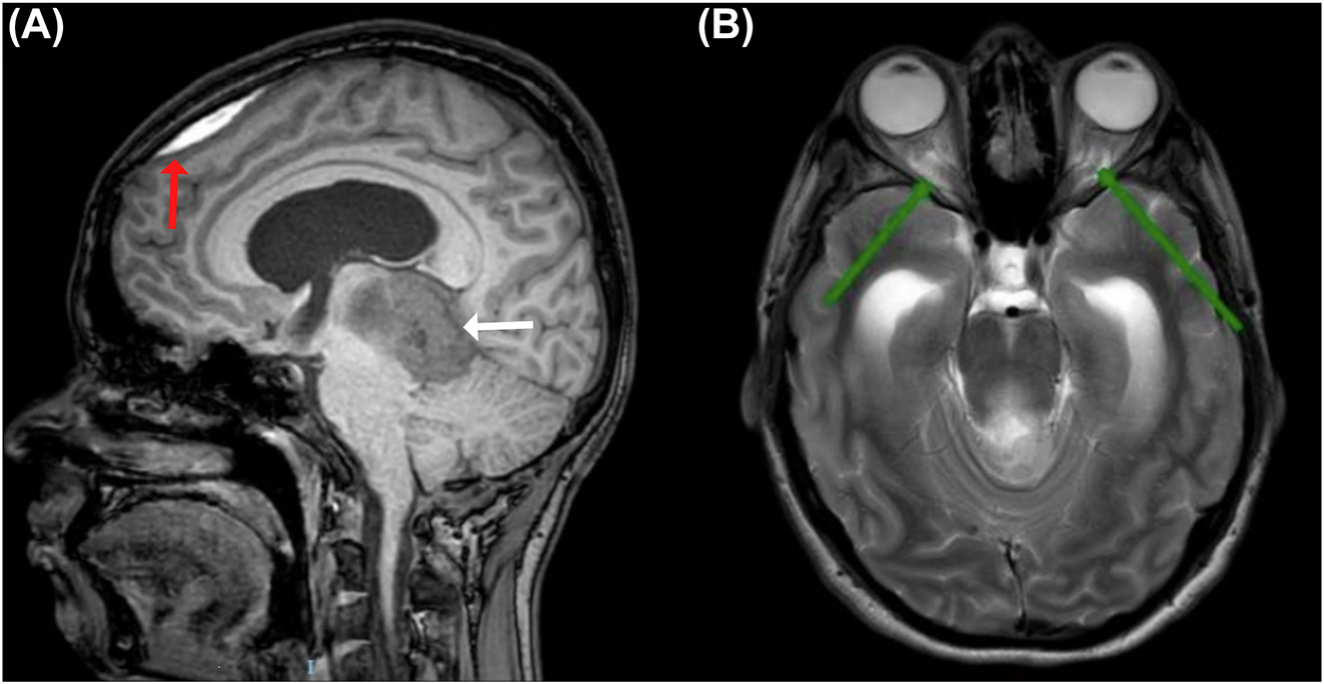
Follow-up brain magnetic resonance imaging. (A) Subdural hematoma adjacent to the frontal bone of 43 mm, with minimal compressive effect on the adjacent frontal parenchyma (red arrow), mass in the pineal region corresponds to ganglioglioma (white arrow) (B) Increase in cerebrospinal fluid around the optic nerves with a tortuous course in the intraconal segment and protrusion of the optic discs (green arrow).
